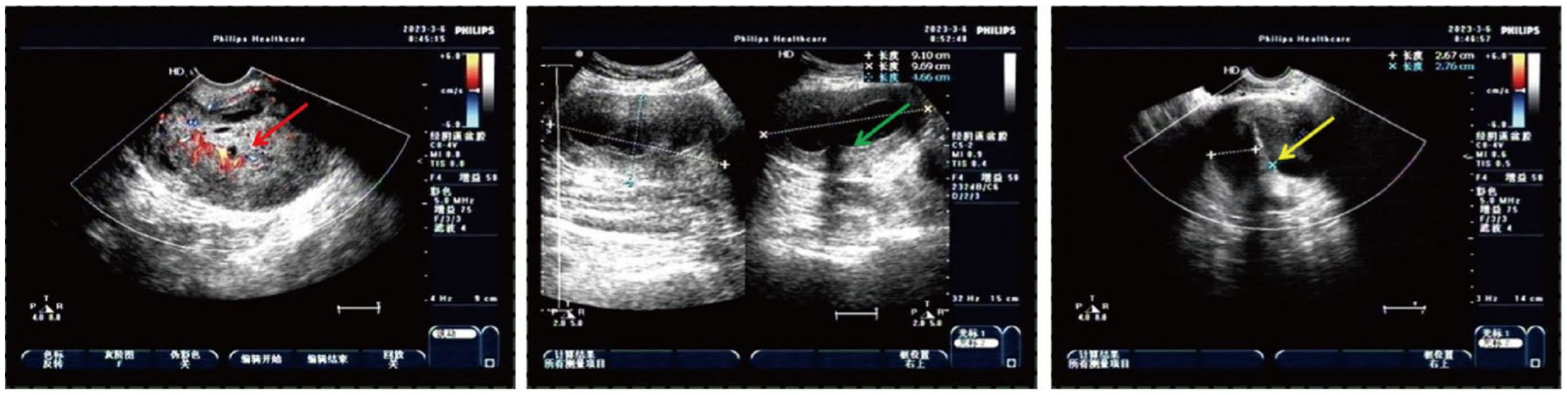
Heterogeneous echo on the left cervical wall and incomplete mediastinal uterus shown by pelvic ultrasound (The red arrow: heterogeneous echo on the left wall of the cervix detected with transvaginal ultrasound; the green arrow: heterogeneous echo on the left wall of the cervix detected with transabdominal ultrasound; and the yellow arrow: incomplete mediastinal uterus).
